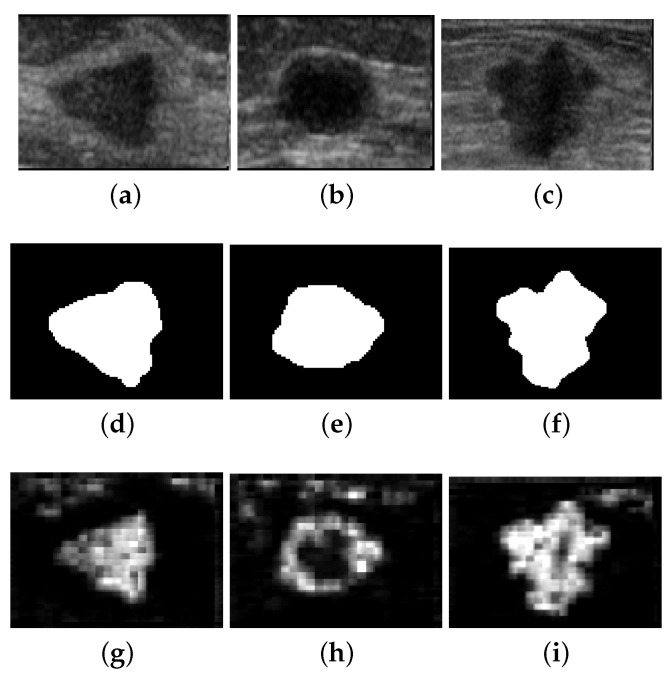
(a–c) Original ultrasound images. (d–f) The segmentation ground-truth. (g–i) The segmentation obtained by CNN after filtering by *q*-sigmoid when β=0.15, α=0.03, λ=1.0, and q=0.1 in Equation (12).
